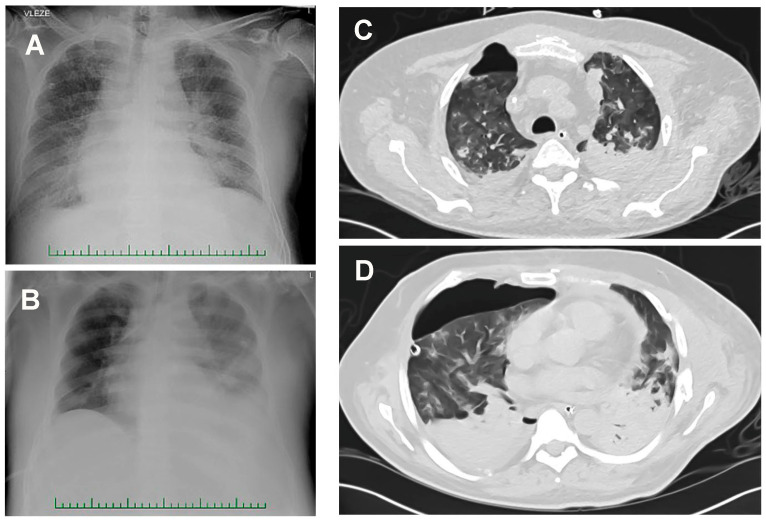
ARF patients’ chest radiography and CT scans. (A,B) Radiographs showing increasing bilateral, inhomogeneous blurring, likely caused by inflammatory infiltrations (Patient 1). (C,D) Atelectasis in both lower lung lobes and irregular multiple ground glass opacities revealed by the CT scan of Patient 2. Right-sided pneumothorax and small amount of fluid in bilateral pleural cavities are also discernable.
